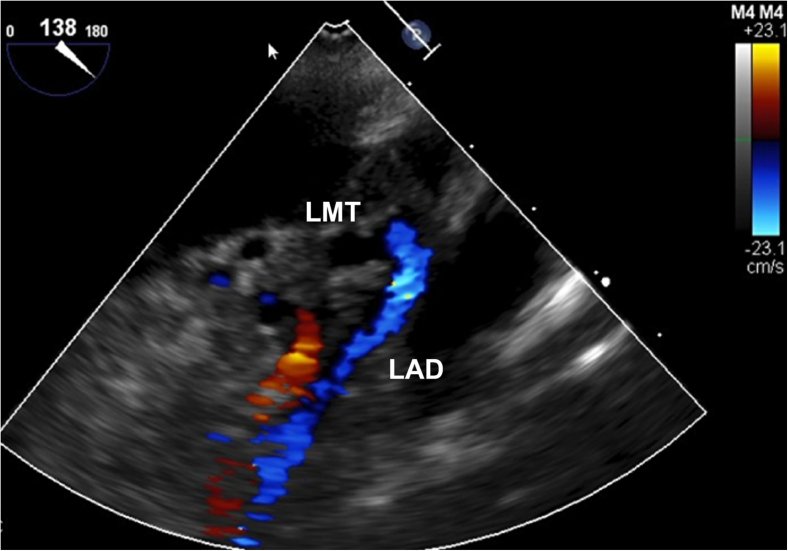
Doppler assessment of cardioplegia delivery. Transesophageal echocardiography with color Doppler shows cardioplegic flow from the left main trunk to the left anterior descending artery during direct left ventricular cardioplegia infusion.
